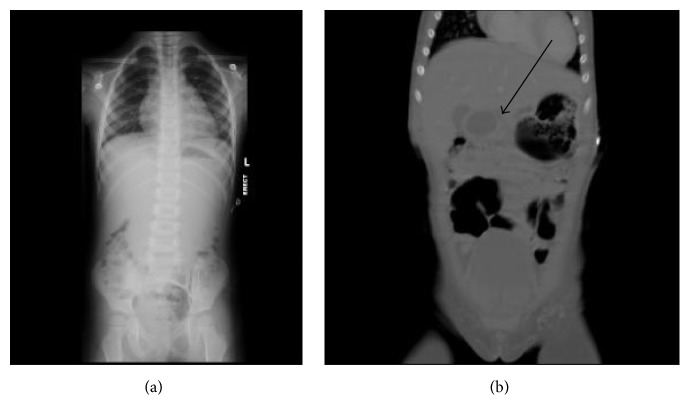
(a) Obstruction series. (b) CT with PO and IV contrast.
